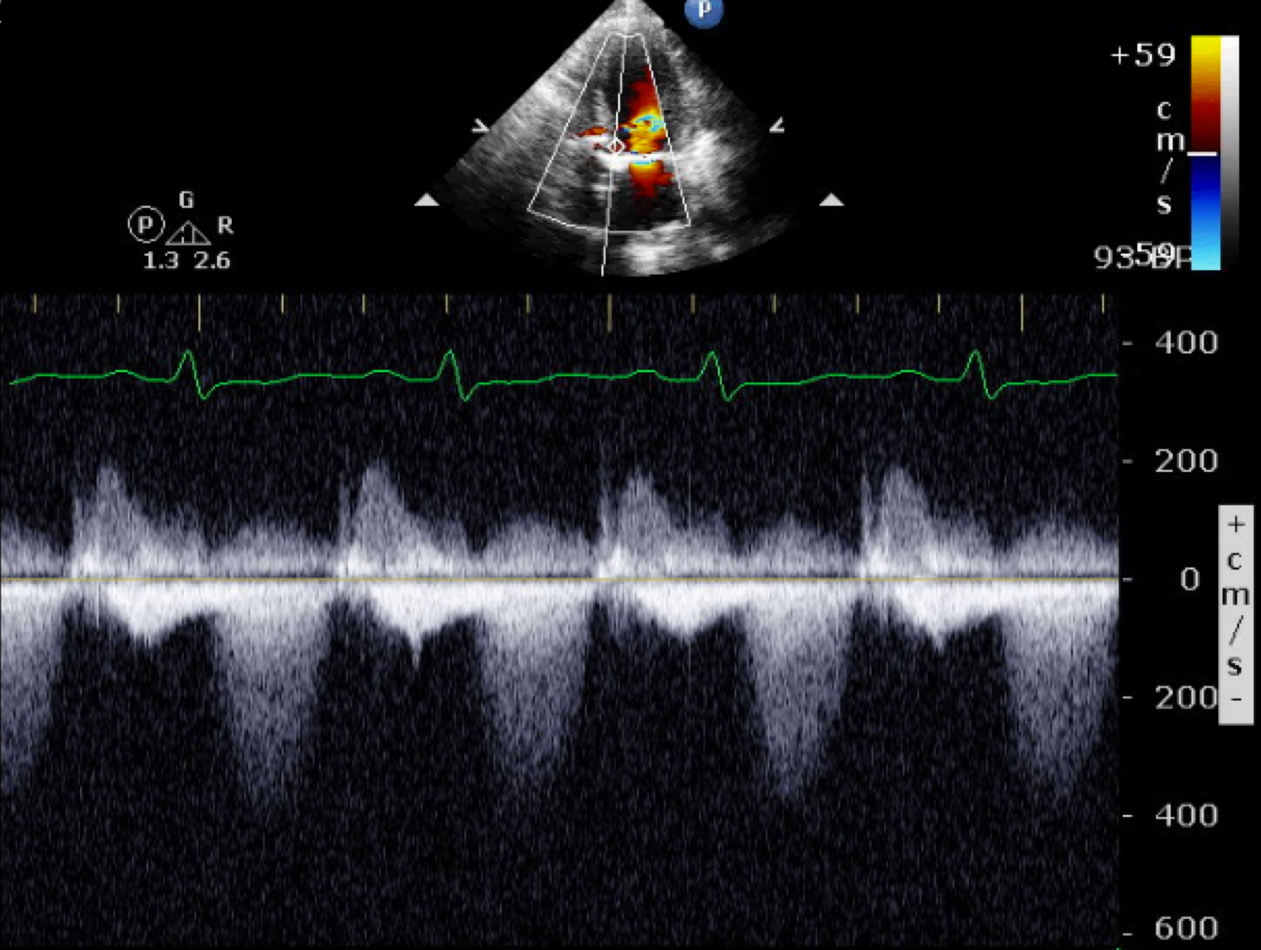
Transthoracic echocardiogram showing continuous wave Doppler signal across the prosthetic aortic valve with a peak velocity of 4.1 m/s.
